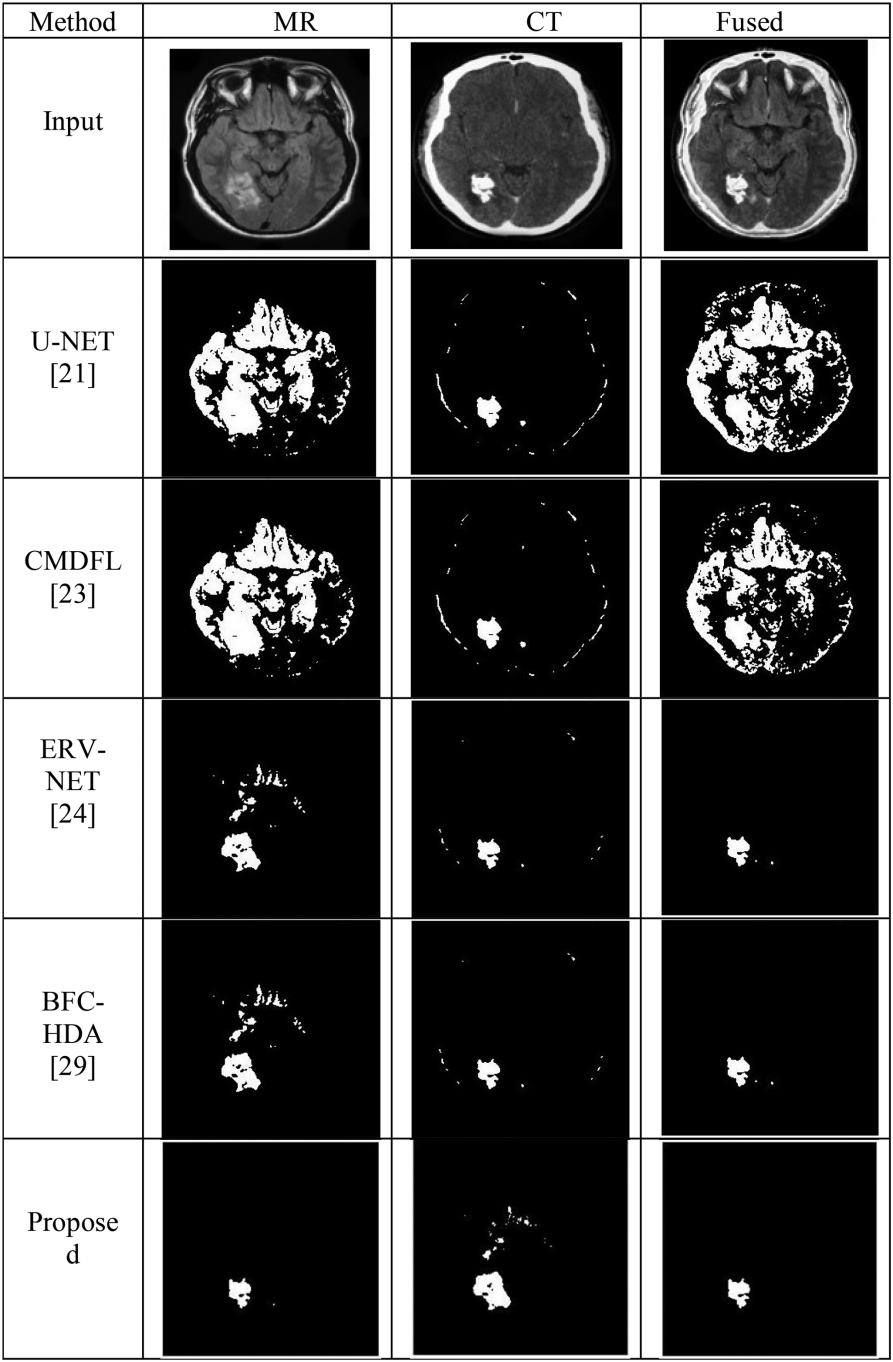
Segmentation performance comparison of MR, CT, and Fused images.
